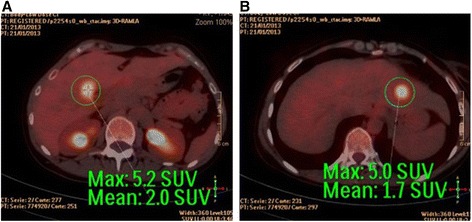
a-b PET-CT images locating the two liver metastases, the first in segment IV-B next to the right portal bifurcation and the other in segment II
